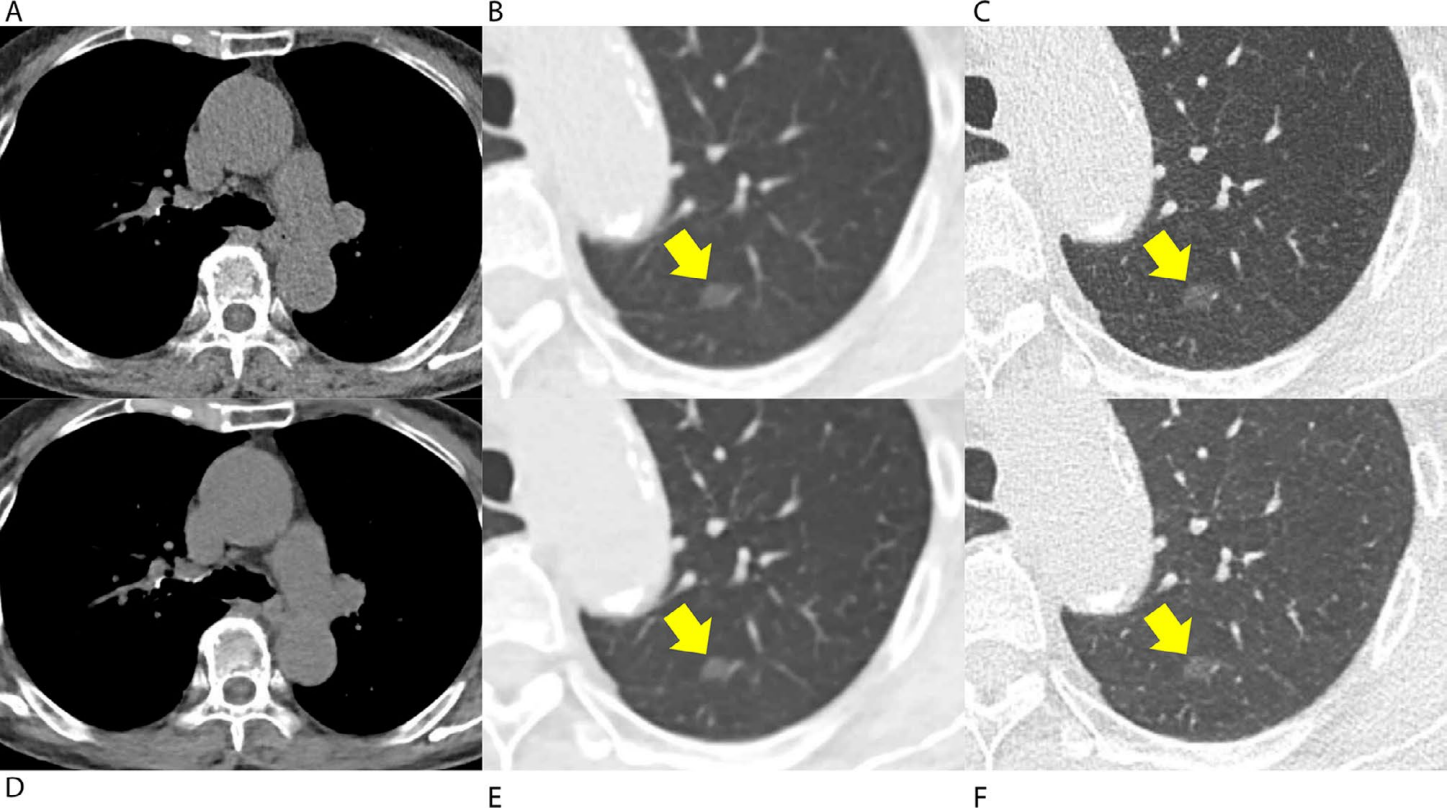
A woman received low-dose chest CT for the follow-up evaluation of a previously detected 8-mm ground-glass nodule in the left upper lobe. (A-C) Conventional low-dose chest CT images were reconstructed with iterative reconstruction, and (D-F) the images generated using a quarter dose of radiation were reconstructed with commercial deep-learning software. (A, D) Soft-tissue structures, including the aorta, subcutaneous fat, and paraspinal muscles, were visualized with a lower noise level in the mediastinal-window setting. (C, F) A ground-glass nodule in the left upper lobe was well visualized with a sharp margin on both images (arrow)
